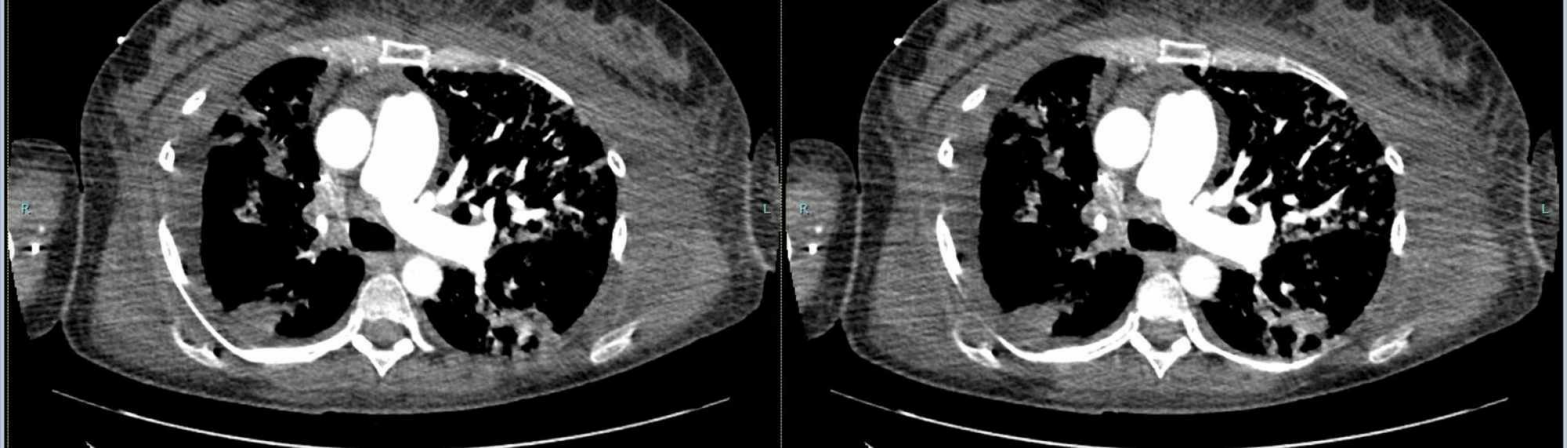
CTA revealed concentric narrowing of the main right pulmonary artery, with pulmonary artery occlusion to the right lower lobe. CTA, CT angiography
